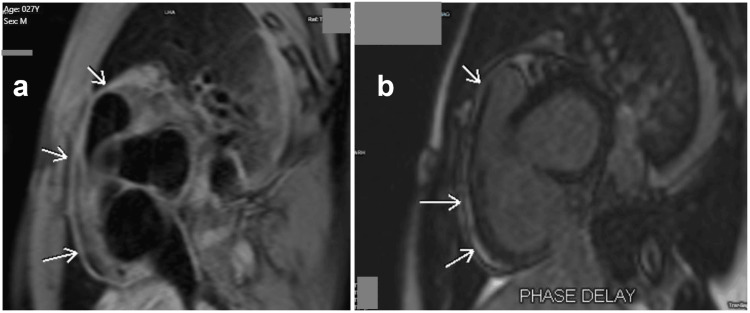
(a) T2-weighted spin echo image depicting the acutely inflamed pericardium which appears high signal (arrow); (b) Late gadolinium enhancement sequence revealing avid uptake of contrast by the inflamed pericardium (arrow)
